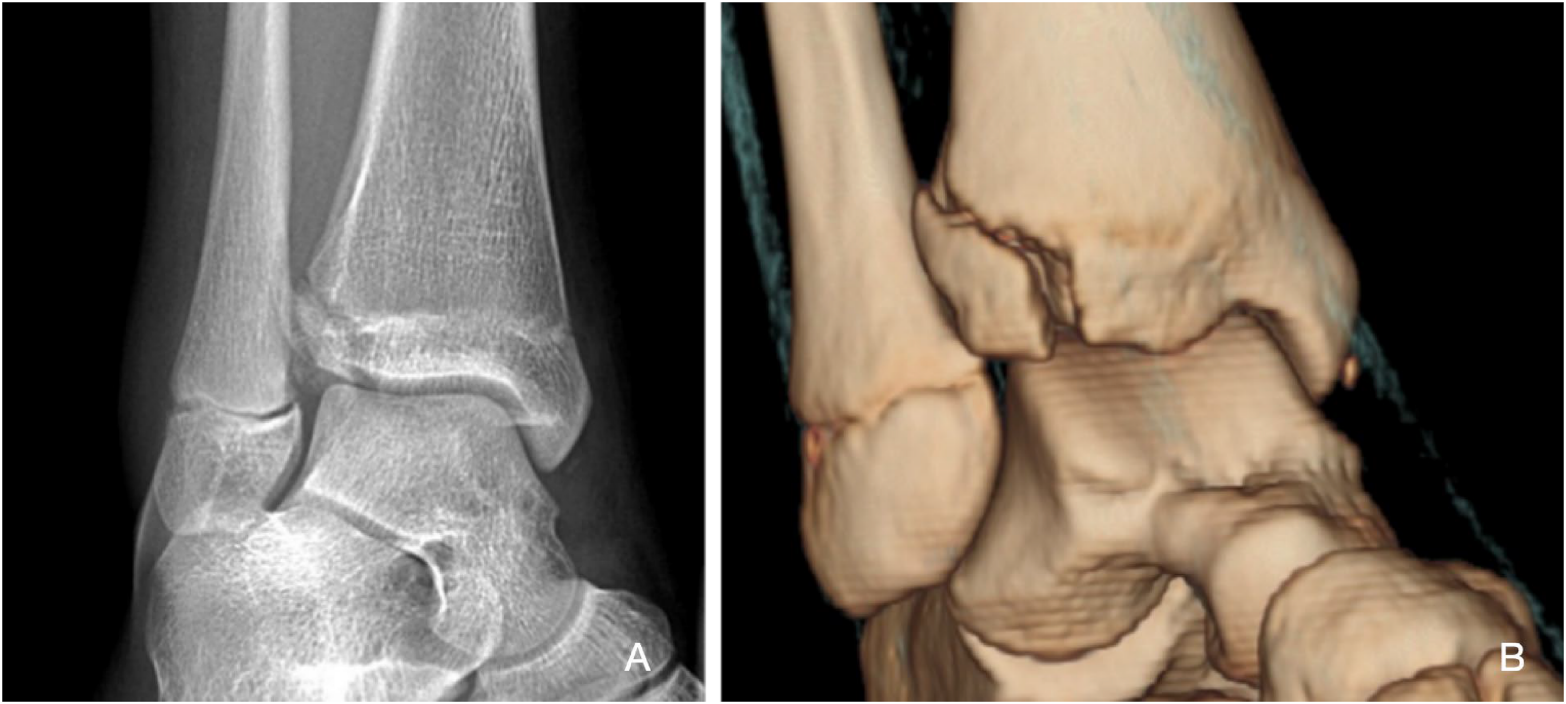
Preoperative x-ray (A) and CT scan (B) of a Tillaux fracture.
